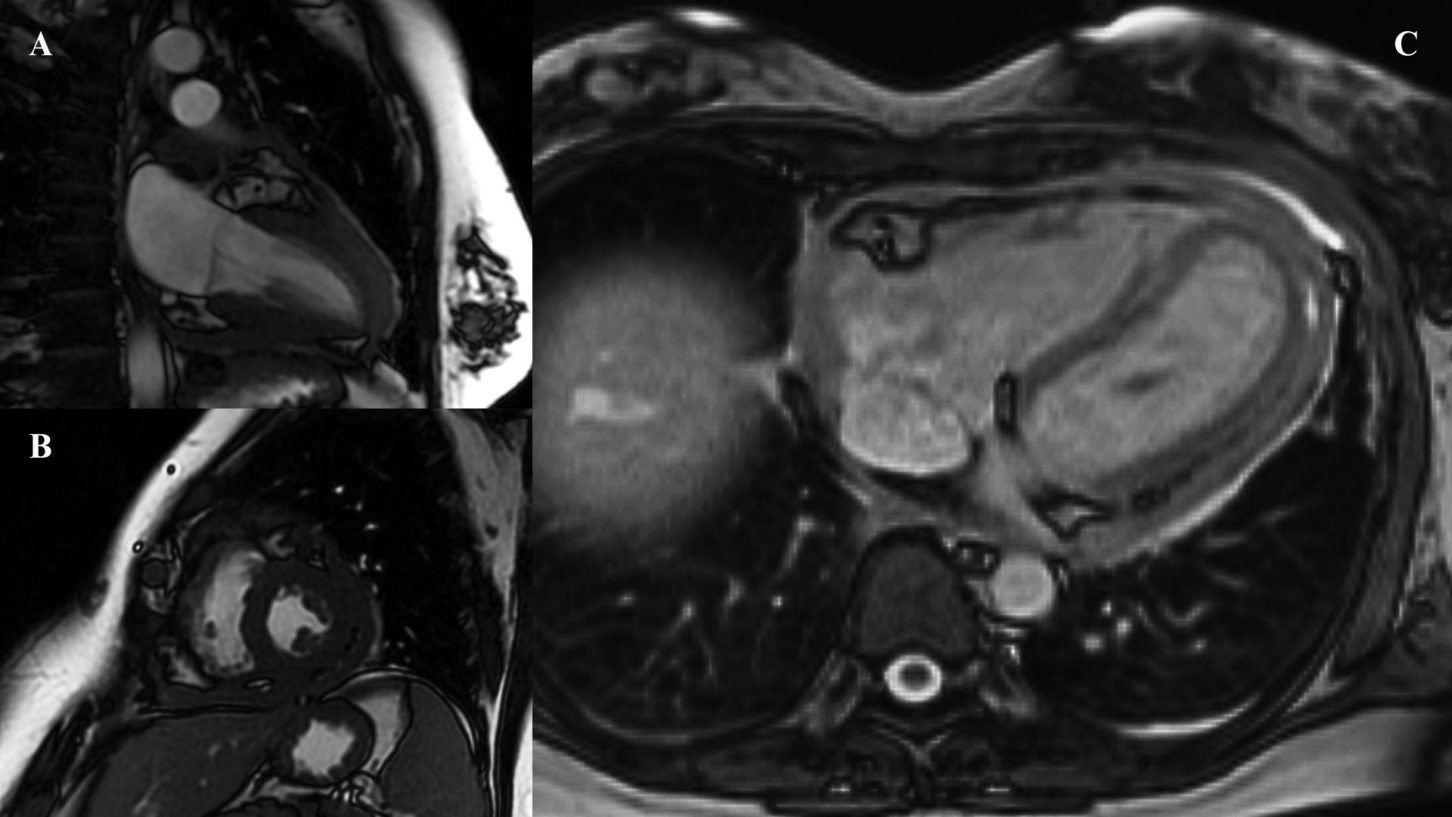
(A, B) First performed MRI scan—short and long axis. (C) MRI performed before pericardiectomy.
